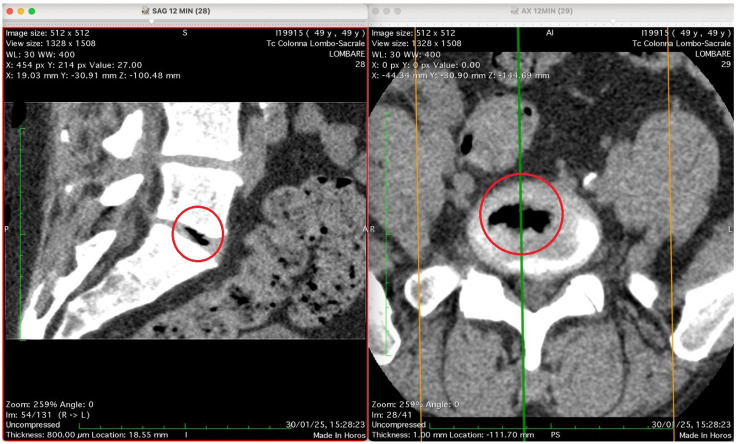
Sagittal (left) and axial (right) CT images of the lumbosacral spine at 12 min post-injection, showing a localized intradiscal gas pocket (red circle), likely at the L4–L5 or L5–S1 level. The gas appears as a linear, low-density area within the central or posterior disc space, aligned with a probable annular fissure. No extension into epidural or paravertebral tissues is seen. Vertebral endplates and neural structures remain intact, with no signs of compression or destructive pathology. Findings are consistent with gas diffusion via annular microfissures following paravertebral oxygen–ozone therapy. Technical details: 512 × 512 matrix, 0.8 mm slice thickness, WL/W: 30/400, scan date: 30 January 2025.
